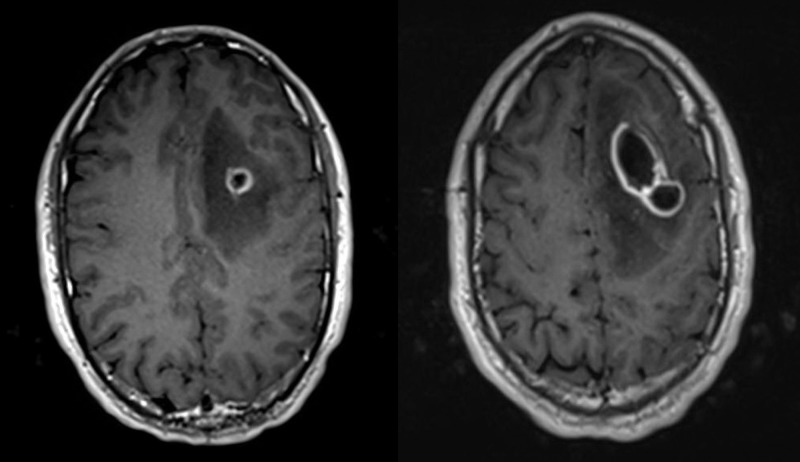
Brain magnetic resonance imaging (MRI) on admission. Brain MRI T1 Axial images showing focal restricted diffusion centered at the left frontal lobe at the grey-white matter junction with surrounding rim enhancement with significant perilesional vasogenic edema with mass effect on the frontal horn of the left lateral ventricle.
